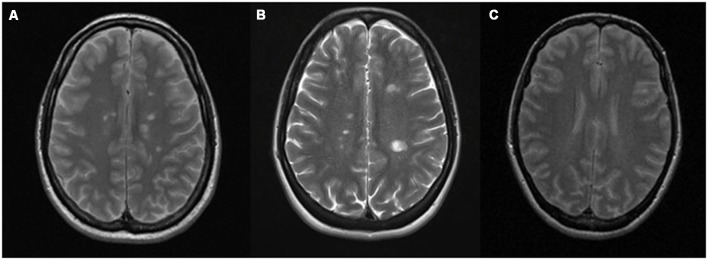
Conventional magnetic resonance imaging (MRI) showing similar demyelinating lesions in: (A) multiple sclerosis (MS) and (B) antiphospholipid syndrome (APS). (A) Gadolinium-enhanced T2-weighted sequence of a patient with MS showing multiple demyelinating lesions in the* supra* and infratentorial white matter, predominantly periventricular, without evidence of postcontrast enhancement. (B) T2-weighted sequence of a patient with APS that shows multiple focal demyelinating lesions in periventricular, juxtacortical posterior left parietal (by confluence of several lesions) white matter. that are hyperintense relative to the normal appearing brain tissue, indicating increased permeability of the blood–brain barrier. (C) Normal T2 MRI.
